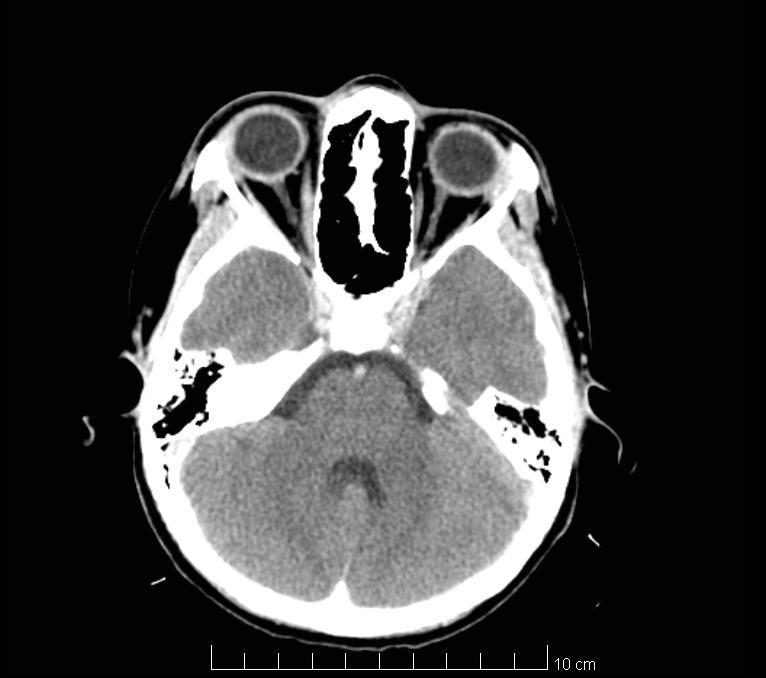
Postoperative Head CT-Scan (blue arrow - orbital cellulitis and osteitis remission; red arrow - sinusitis remission)
